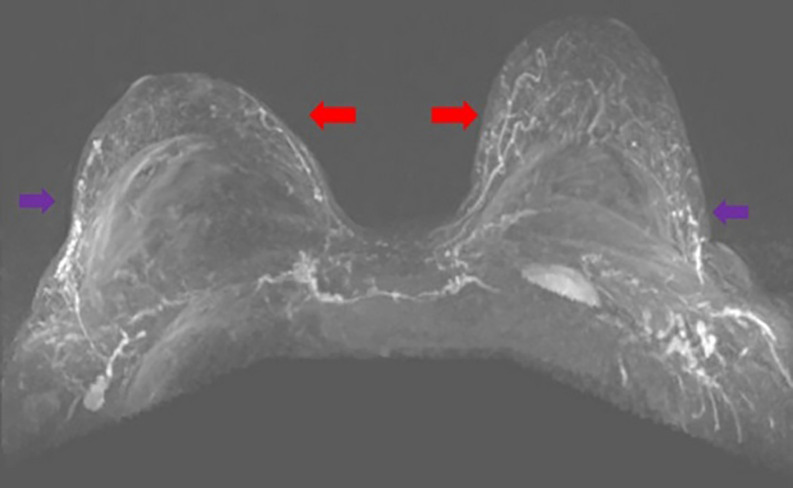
Axial MRI maximum intensity projection (MIP) images. The pectoralis major perforators originating from the internal mammary vessels (red arrows). The vessels can be traced heading toward the nipple areola complex. The lateral vessels (purple arrows) do not quite reach the nipple areola complex on either side. Therefore, in this case, the medial blood supply was selected to keep the nipple areola complex alive.
